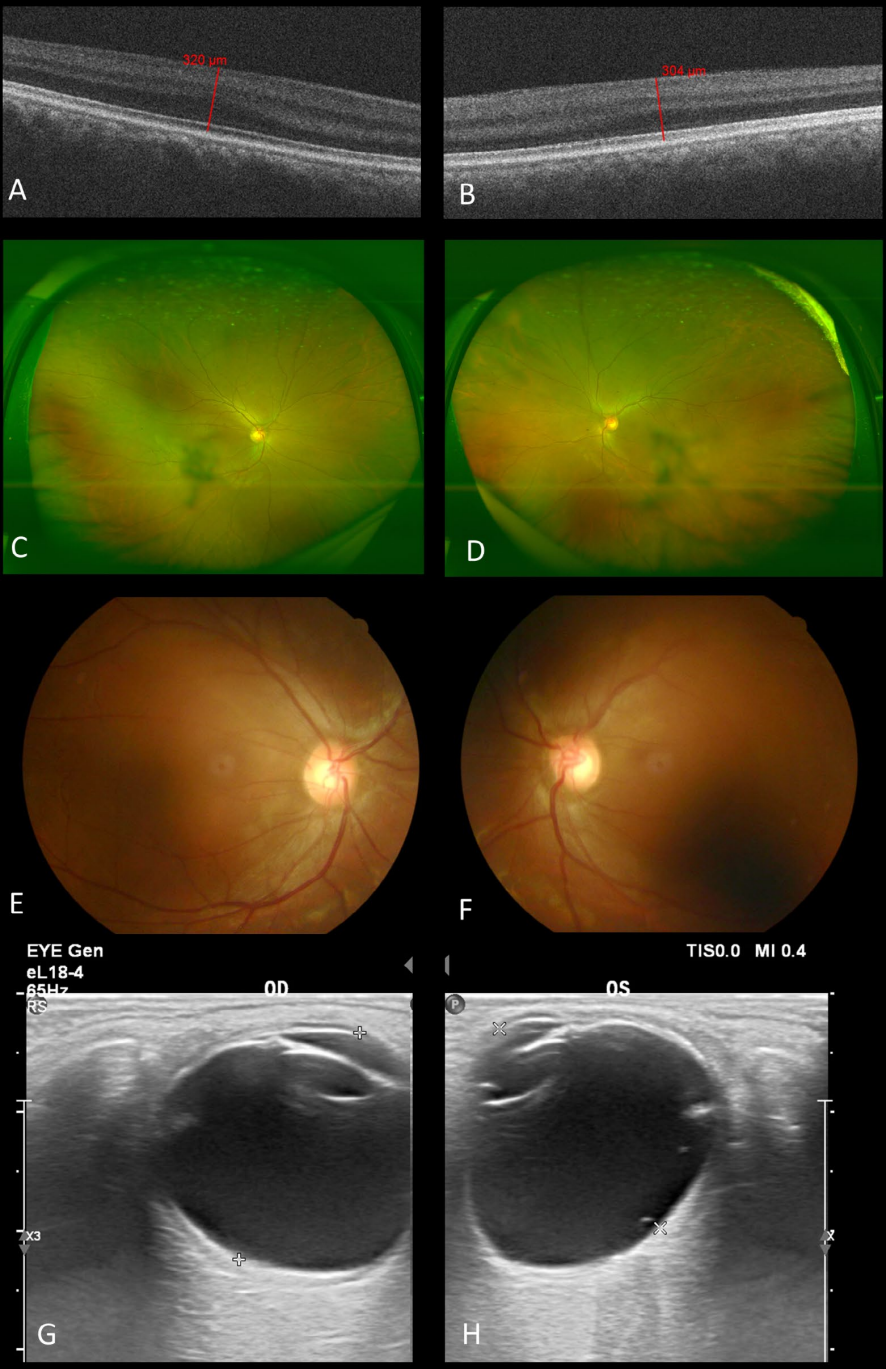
Posterior segments of the mother. (Left column for the right eye and right column for the left eye.) A-B. Optical coherence tomography of the macula. Both eyes exhibited grade 3 FH with only ONL widening, foveal retinal thickness measuring 320 μm and 304 μm respectively. C-F. Bilateral opacity of the vitreous and cupping of the left optic nerve head (cup/disc ratio about 0.5) was presented. The trunk of nasal retinal vein divided into upper and lower branches only after crossing the edge of optic disc. G-H. Ophthalmic ultrasound showed bilateral vitreous opacity and the axial length was 21.9 mm (right eye) and 21.8 mm (left eye). No space-occupying lesions or retinal detachment was observed
